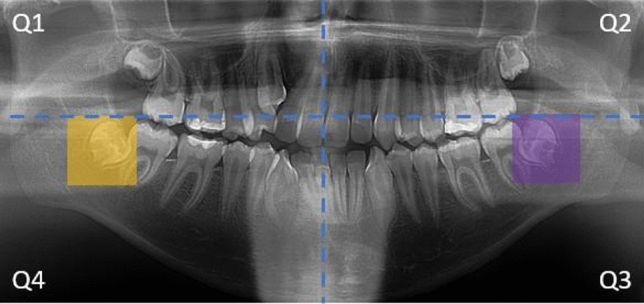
A panoramic X-ray image. Q3 and Q4 wisdom teeth are highlighted.
